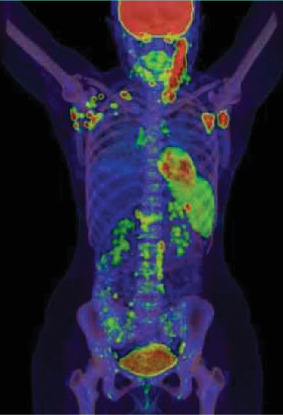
A coronal whole-body PET-CT demonstrating multiple areas of increased metabolic activity. There is intense FDG uptake involving lymph nodes both above and below the diaphragm, consistent with widespread nodal involvement. Prominent heterogeneous FDG uptake is observed in the bone marrow, which may suggest marrow activation or infiltration. Additionally, there is an intensely hypermetabolic focus located immediately posterior to the right kidney.
